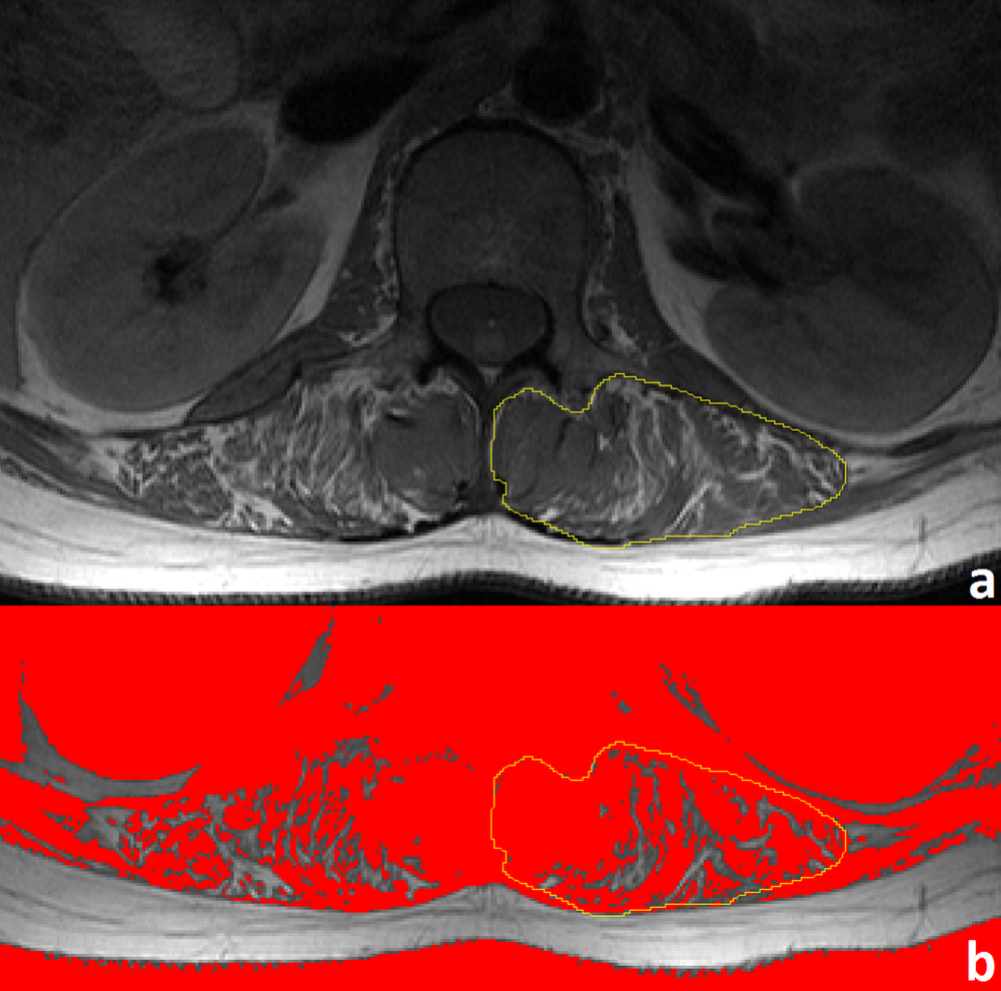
MR-derived measurement of the fat fraction. A region of interest is selected from a T1-weighted TSE sequence in the left paraspinal musculature at the L3-level (panel a). Fat fraction is calculated using the equation FF = 1 –FCSA/CSA; a signal intensity threshold of 772 (derived from normal muscle–the medial multifidus muscle in this case) segregates muscle from fat tissue. Pixels beneath this threshold, colored red (panel b), constitute the FCSA (1096 mm^2^), while the entire area of the ROI constitutes the CSA (1466 mm^2^). These data result in an FF value of 0.25.
